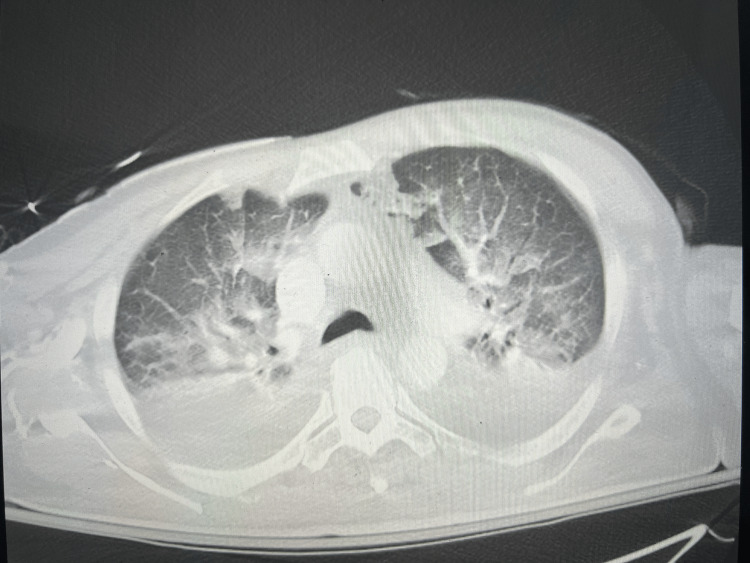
CT angiography pulmonary embolism study showed bilateral confluent airspace disease concerning for infection and moderate-sized pleural effusions bilaterally. No evidence of pulmonary embolism was noted.
